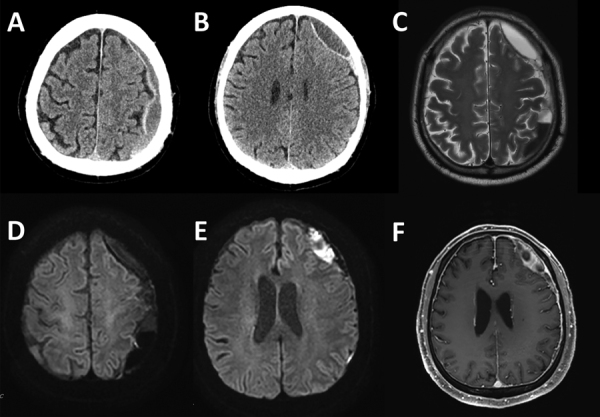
Initial image findings of subdural empyema in a patient with *Streptococcus suis* infection, Jeju Island, South Korea. A, B) Computed tomography scans. D–F) Magnetic resonance imaging: diffusion weighted (D, E), T2 (C), and enhanced T1 (F).
